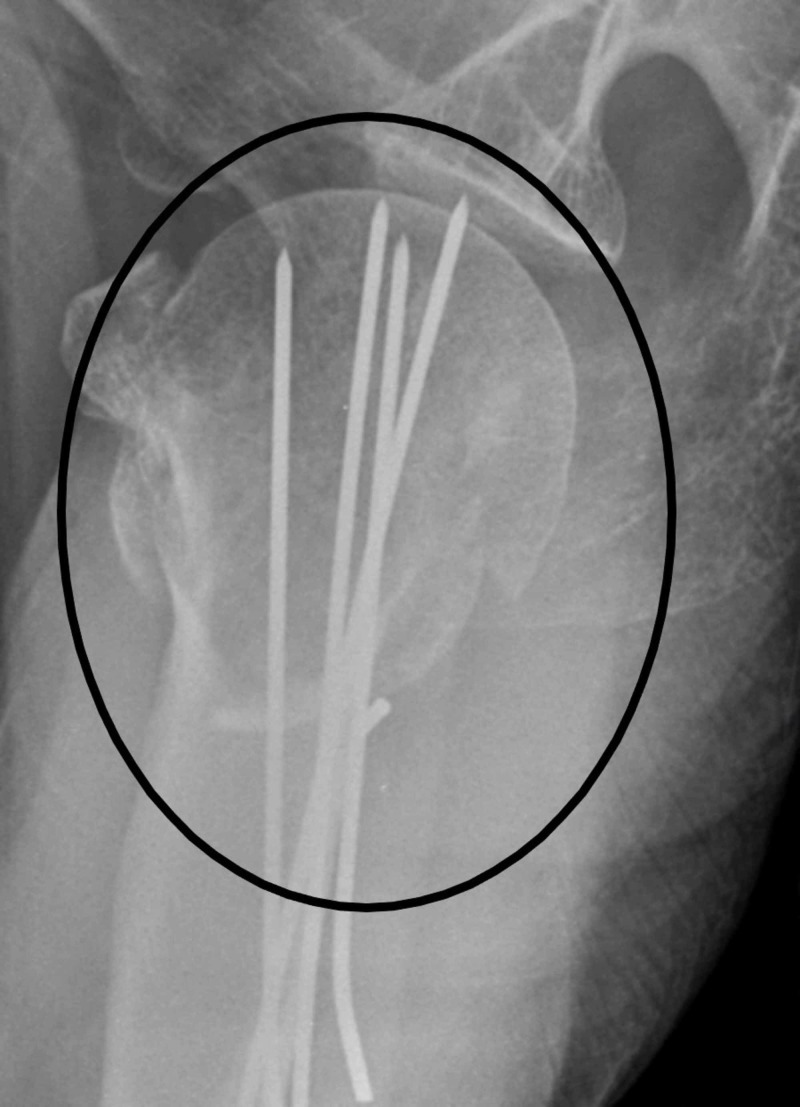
Lateral X-ray view of fixation (encircled)
